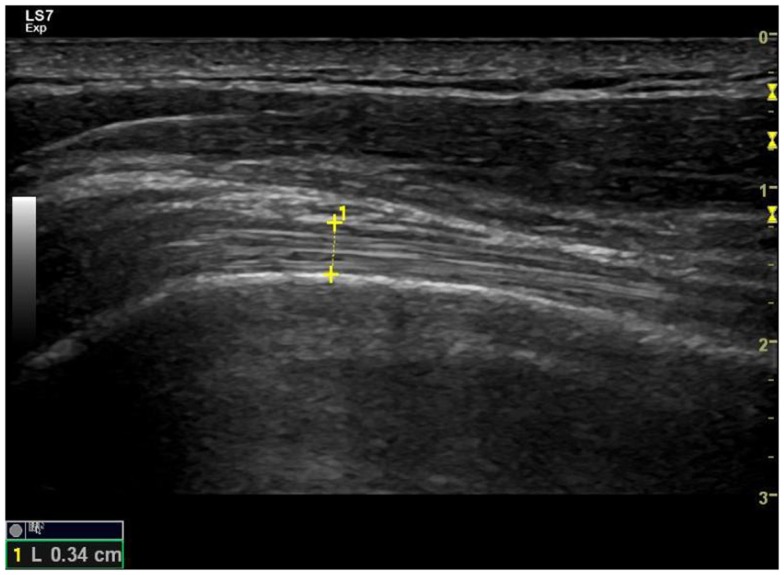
Long-axis ultrasound examination which shows the fibrillar pattern of the long-biceps tendon.
